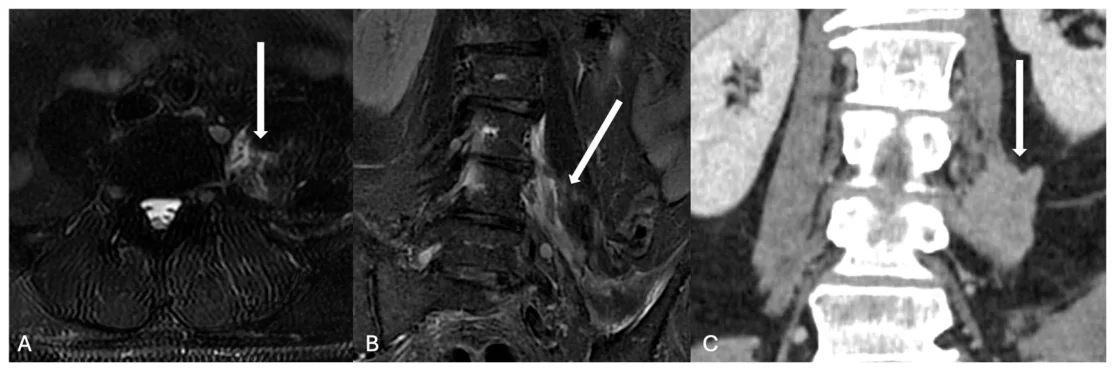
Case 4. A 66-year-old man presented with progressive left-sided low-back and groin pain radiating to the anterior thigh, associated with paresthesia and mild proximal lower-limb weakness. Axial fat-suppressed T2-weighted MRI (A) and contrast-enhanced fat-suppressed T1-weighted MRI (B) demonstrate infiltrative fibro-inflammatory tissue extending through the left paravertebral and iliopsoas compartments and encasing the lumbar plexus (white arrows). Coronal contrast-enhanced CT (C) confirms the retroperitoneal extension of the lesion. The final diagnosis was IgG4-related fibro-inflammatory disease. Original Image.
